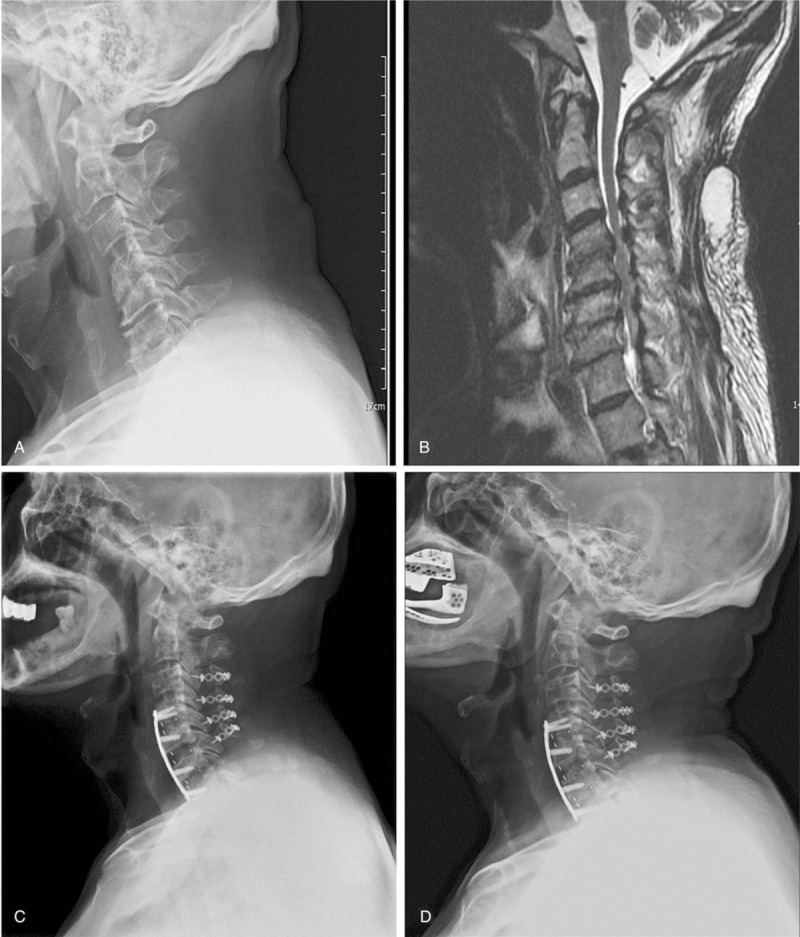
A 65-year-old man underwent C3-6 EOLP and C7 partial laminectomy followed by C4-7 ACDF with PEEK cages. (A) The preoperative plain film demonstrated CKD. (B) Midsagittal T2-weighted MRI revealed C3-7 multilevel stenosis with spinal cord compression. (C) The postoperative 3-month plain film revealed restoration of the cervical lordotic curve and enlargement of the spinal canal. (D) The postoperative 12-month plain film revealed an effective union of the anterior fusion structure. ACDF = anterior cervical discectomy fusion, CKD = cervical kyphotic deformity, EOLP = expansive open-door laminoplasty, MRI = magnetic resonance imaging, PEEK = polyetheretherketone.
